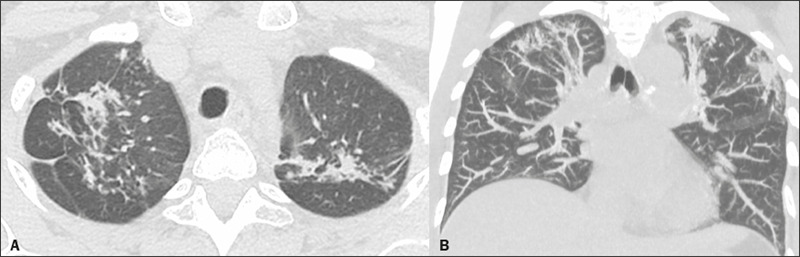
Follow-up CT scan of the patient in Figure 2, performed three months
